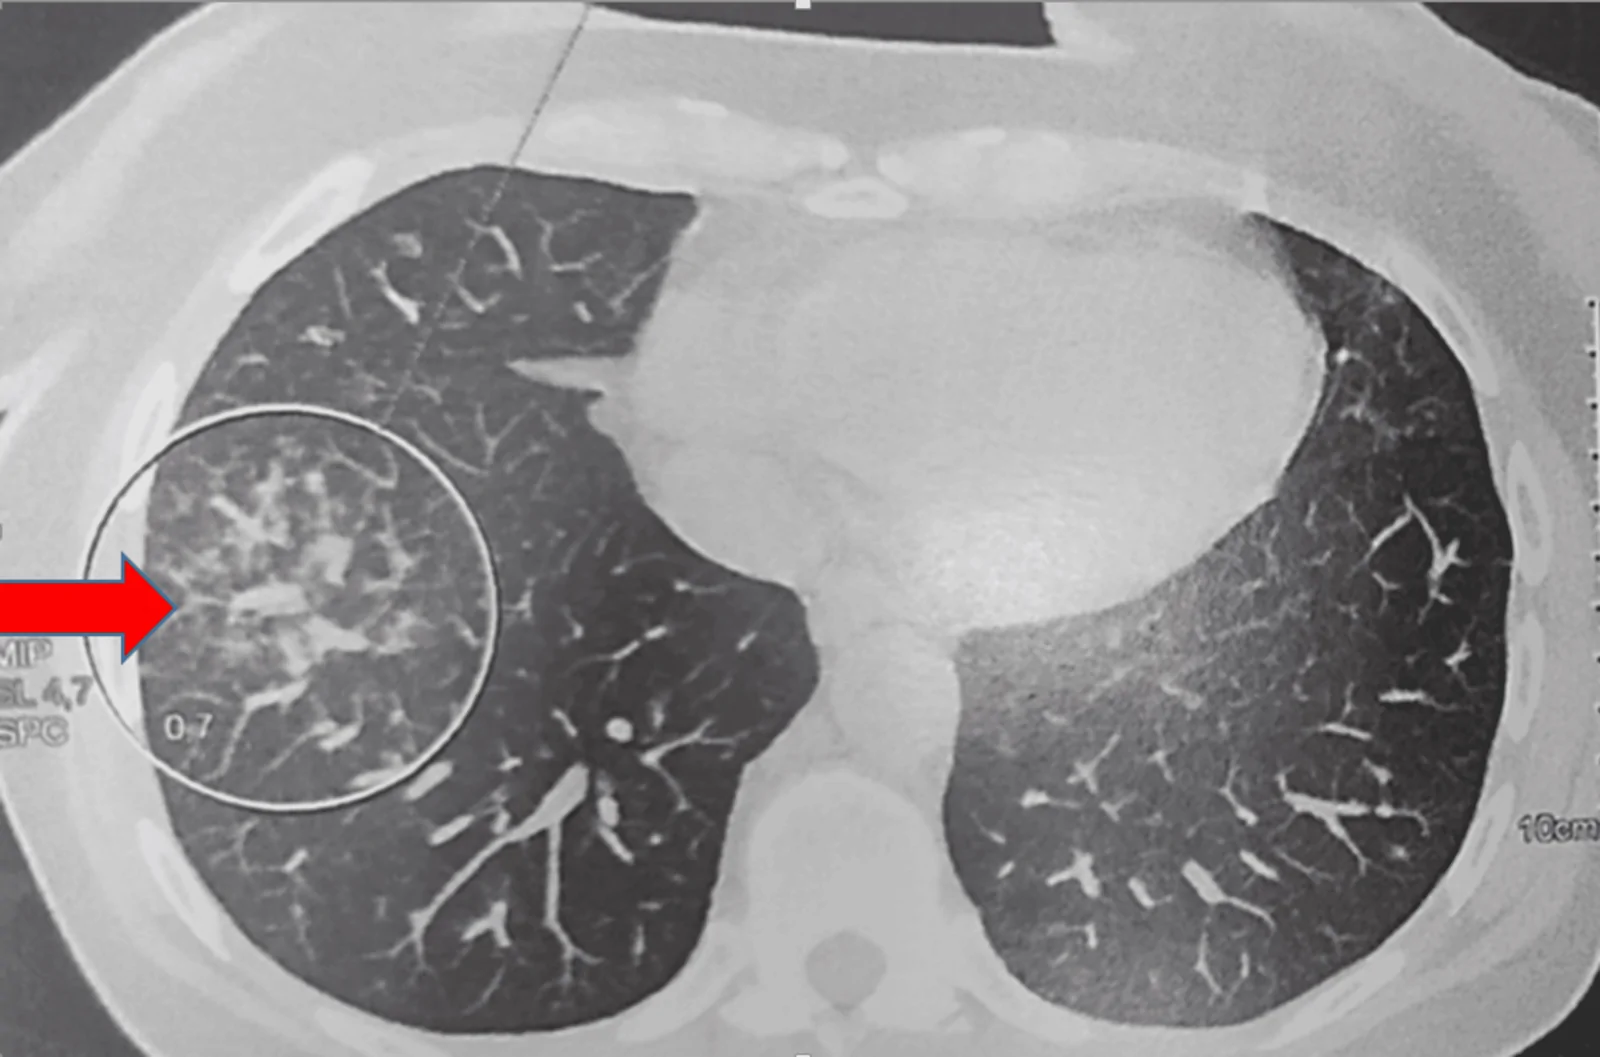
Initial chest computed tomography scan (2024) Right-sided pneumonic consolidation associated with centrilobular tree-in-bud micronodules (red arrow)
